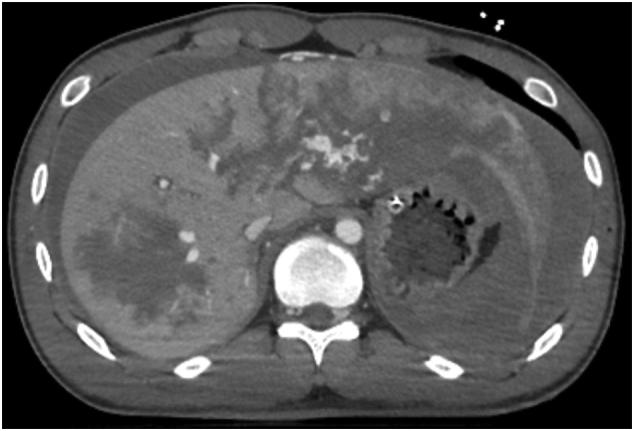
Enhanced abdominal computed tomography showing abdominal bleeding and left and right liver lobe injuries.
